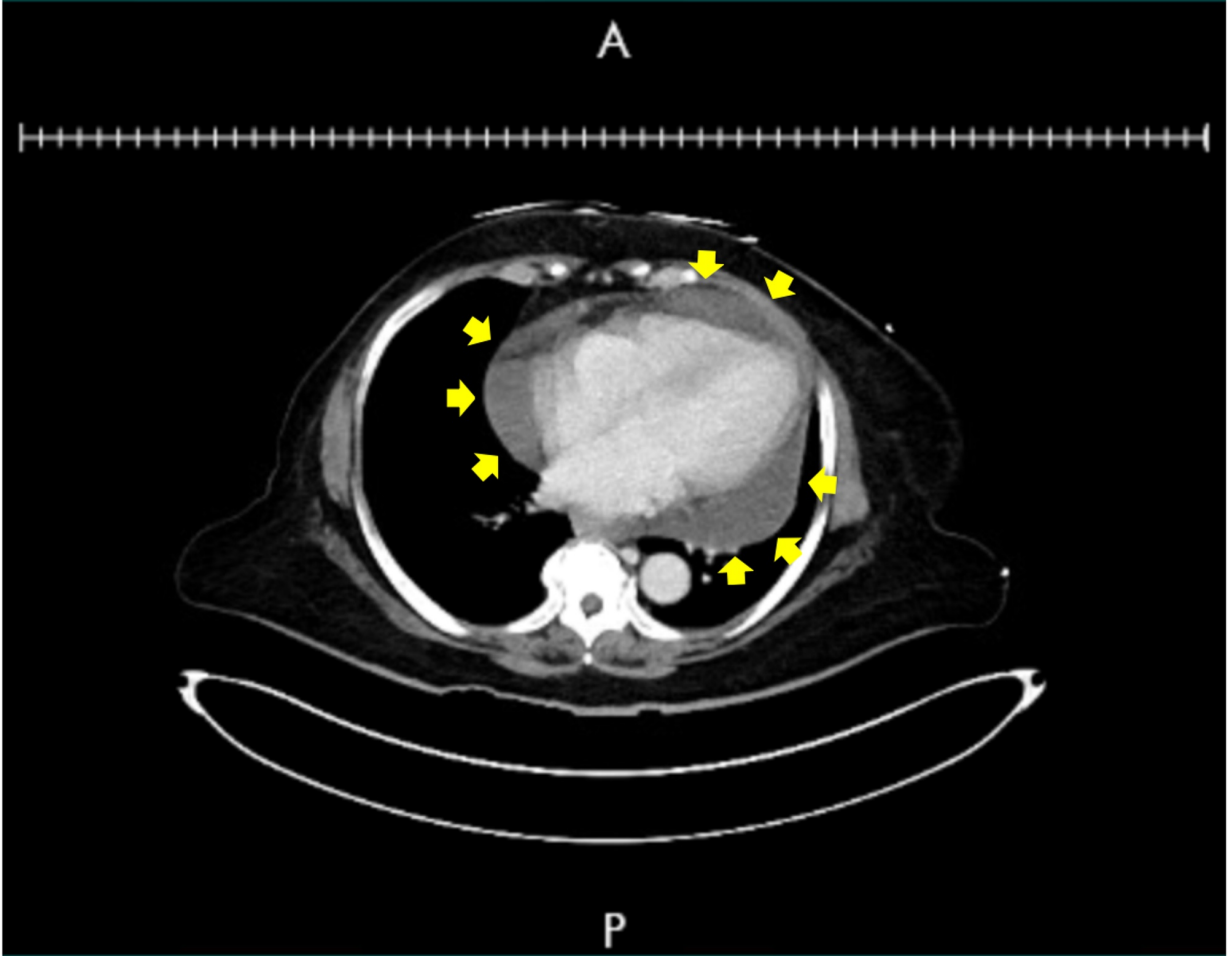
Computed tomography image The image demonstrates large pericardial effusion (yellow arrows denote outline of pericardial effusion surrounding cardiac silhouette)
